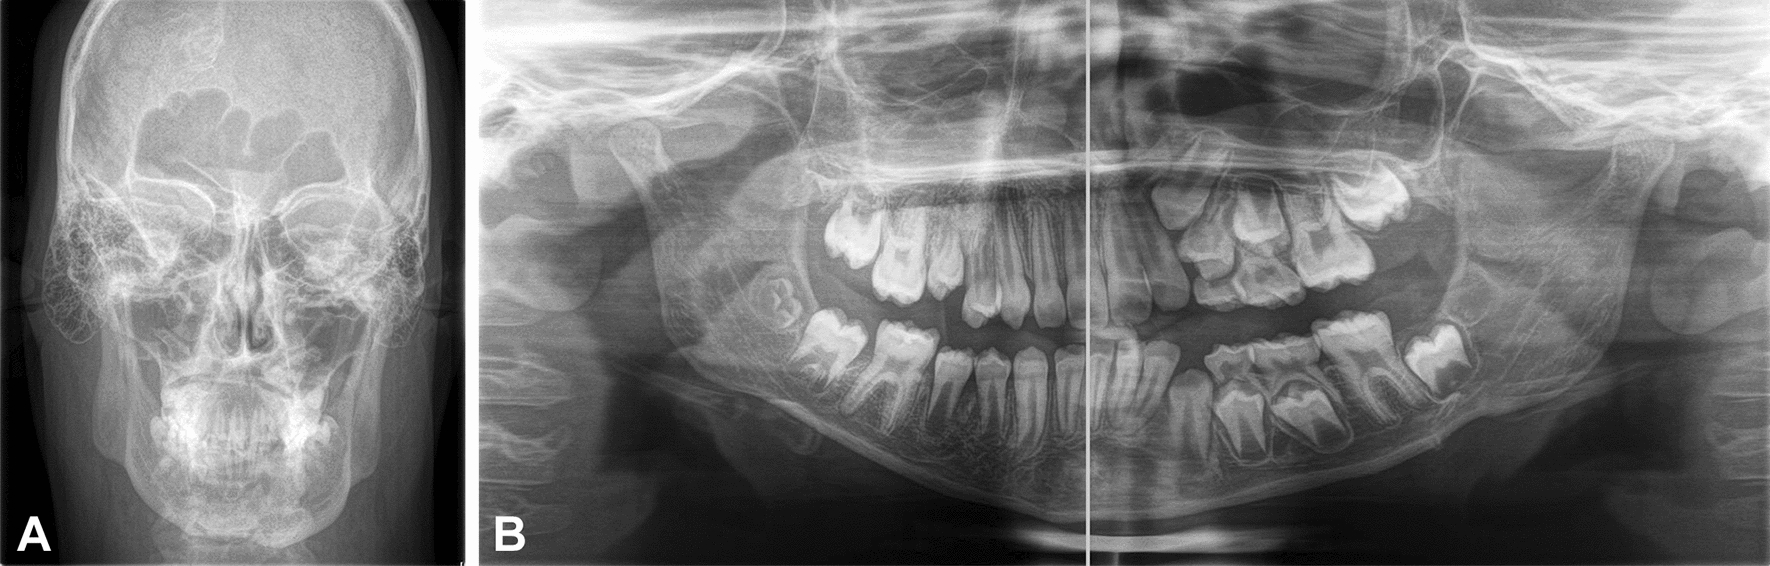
X-ray images of two patients affected with Sturge–Weber syndrome. In A the skull antero-posterior view of an adolescent shows asymmetry of the frontal sinus, petromastoid region of the temporal bone, and mandibular inferior margin. B Dental panoramic radiography of an 8-year-old child with a right hemifacial capillary malformation. Note the asymmetry in deciduous tooth replacement between the right and left side
